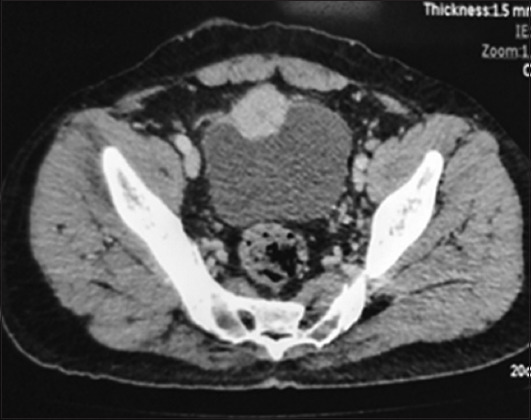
Contrast-enhanced CT showing a hyperdense mass along the anterior wall of the urinary bladder. Abbreviations: CT: Computed tomography.
